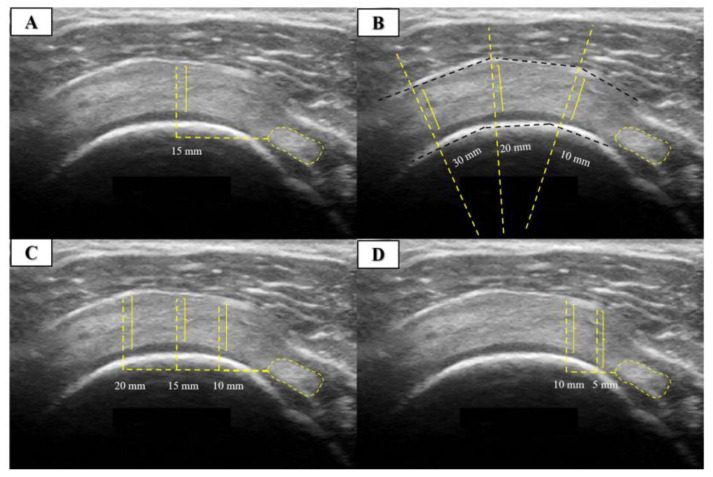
Ultrasound measurement of the supraspinatus tendon thickness in short axis. (A) Measurements were taken at 15 mm [5], (B) at 10, 20 and 30 mm [13], (C) at 10, 15 and 20 mm [6], and (D) at 5 and 10 mm [4] lateral to the most hyperechogenic reference point of the biceps tendon.
